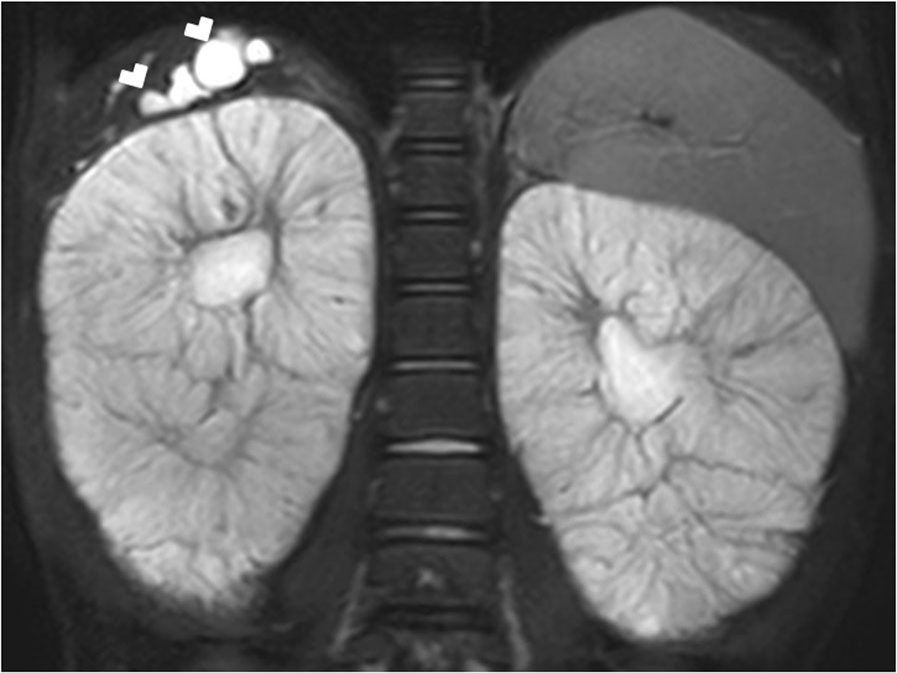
A 5-year-old male patient with known ARPKD. Coronal plane T2W MR image shows enlarged kidneys with diffusely increased T2 signal. Also note subcentimeter liver cysts (arrowheads)
